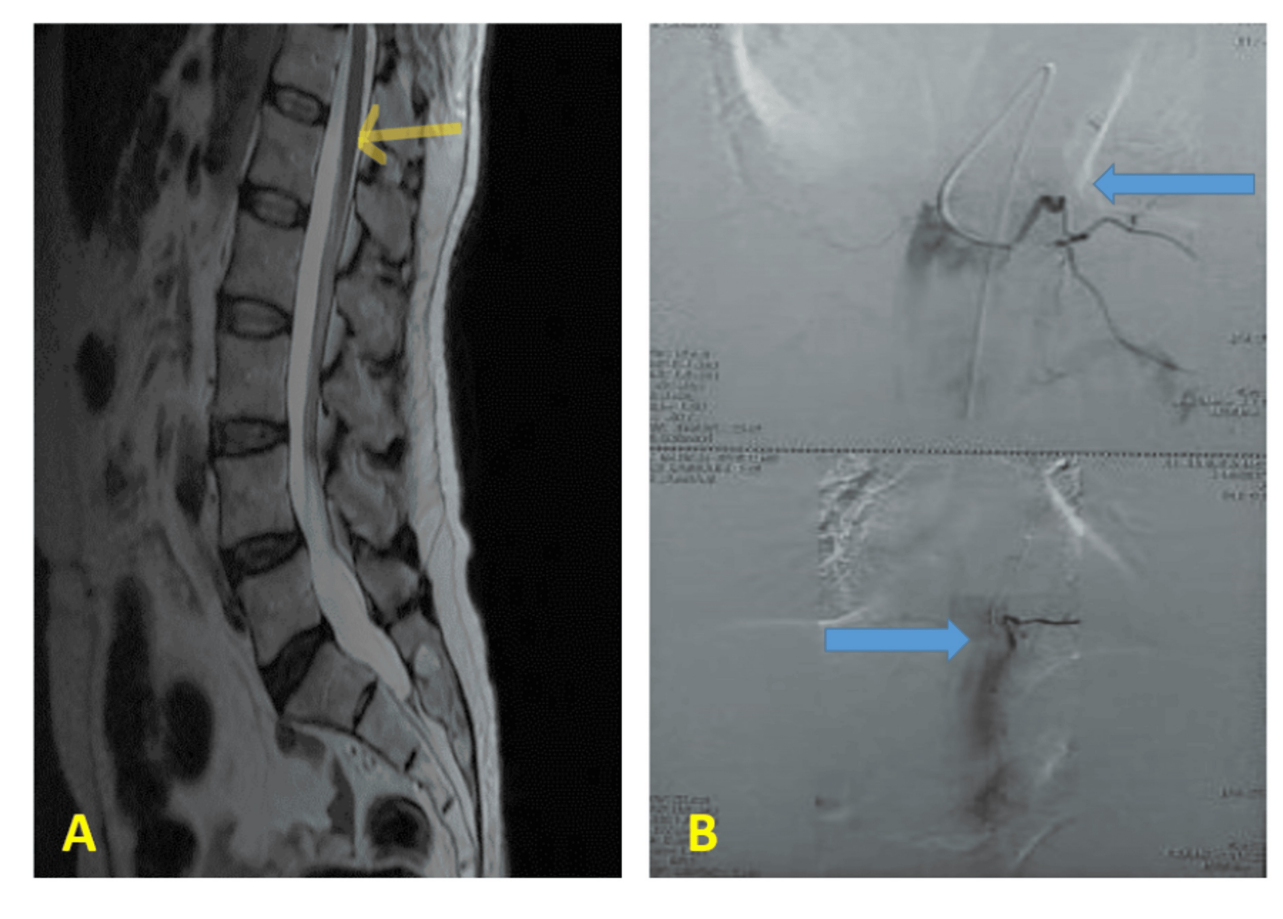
Postoperative spinal MRI and spinal angiography. (A) Postoperative spinal MRI: T2-weighted sagittal sequence control spinal MRI that shows spinal cord hypersignal regression (yellow arrow) and the disappearance of flow void around the spinal cord. (B) Postoperative spinal angiography shows the complete exclusion of the dural fistula and the disappearance of serpiginous venous dilatations in the perimedullary vein (blue arrows).
